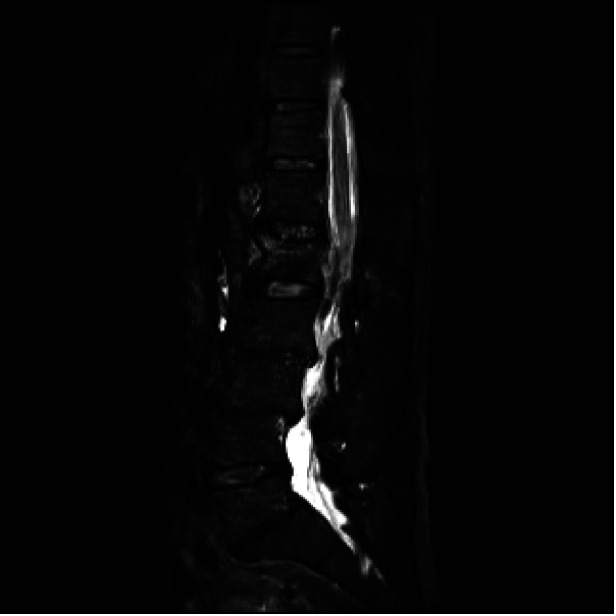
MRI showing fresh compression fracture of L1 vertebrae.
